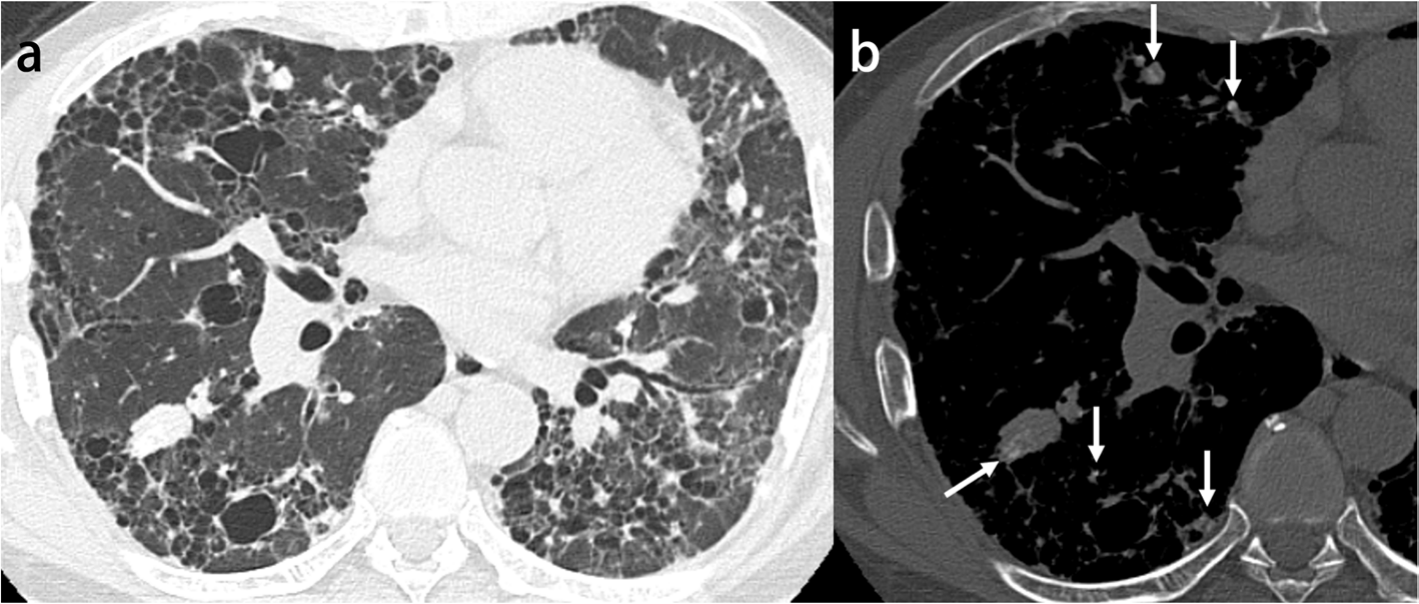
Pulmonary hyalinizing granuloma in a 47-year-old man. a CT image shows multiple bilateral irregular well-circumscribed nodules. Cyst formation is also seen. b Bone window image shows calcification in the nodules (arrows)
